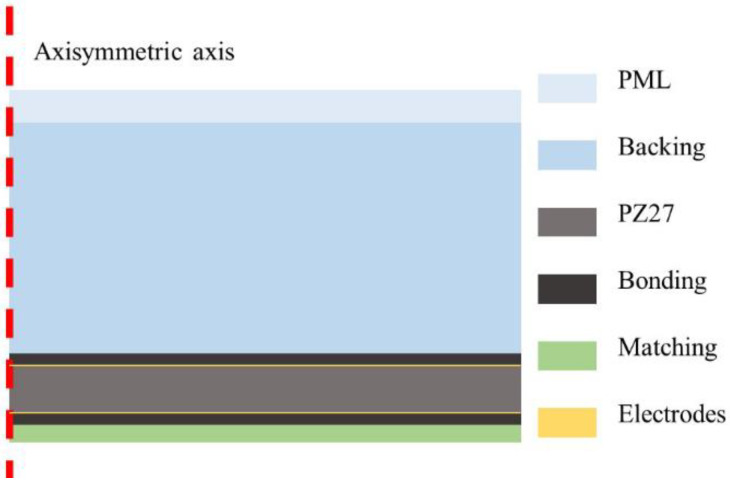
2D axisymmetric FE model of an intact single-element ultrasonic transducer.
